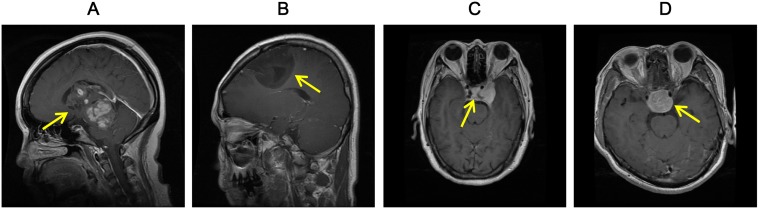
Four examples of brain tumors in T1-weighted CE-MR Images. The tumors are indicated by the yellow arrows in each image. (A) and (B) Gliomas in different subjects having dissimilar appearances. (C) A meningioma and (D) a pituitary tumor from different subjects showing a similar appearance.
